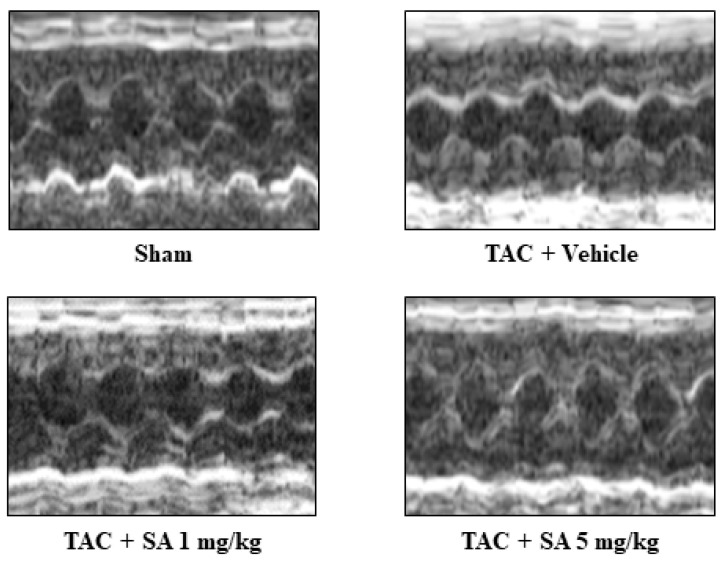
Sarpogrelate suppressed TAC-induced cardiac hypertrophy and systolic dysfunction in vivo. Eight weeks after TAC surgery, cardiac hypertrophy and cardiac function were assessed by echocardiography. Representative images of echocardiography from sarpogrelate- and vehicle-treated TAC and sham are shown.
